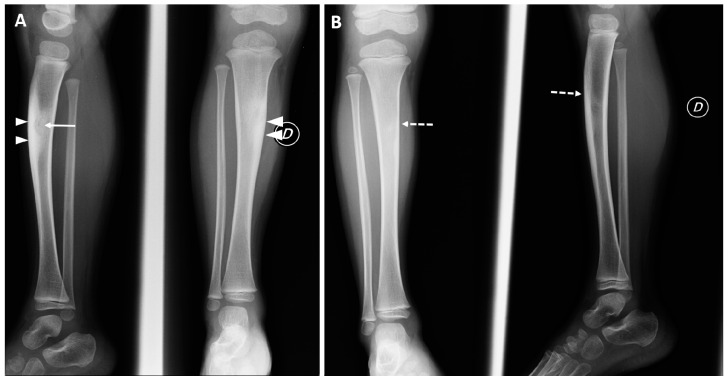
Conventional radiographs of a 35-month-old male (at the time of the treatment), performed after 2 months (Panel (A)) and 18-month follow-up (Panel (B)). Cortical thickening, mild tibial shape deformity (arrowheads), and the presence of the nidus (arrow) were still detectable at first control. Complete normalization of radiographic findings (dotted arrows) were assessed at the second follow-up control.
